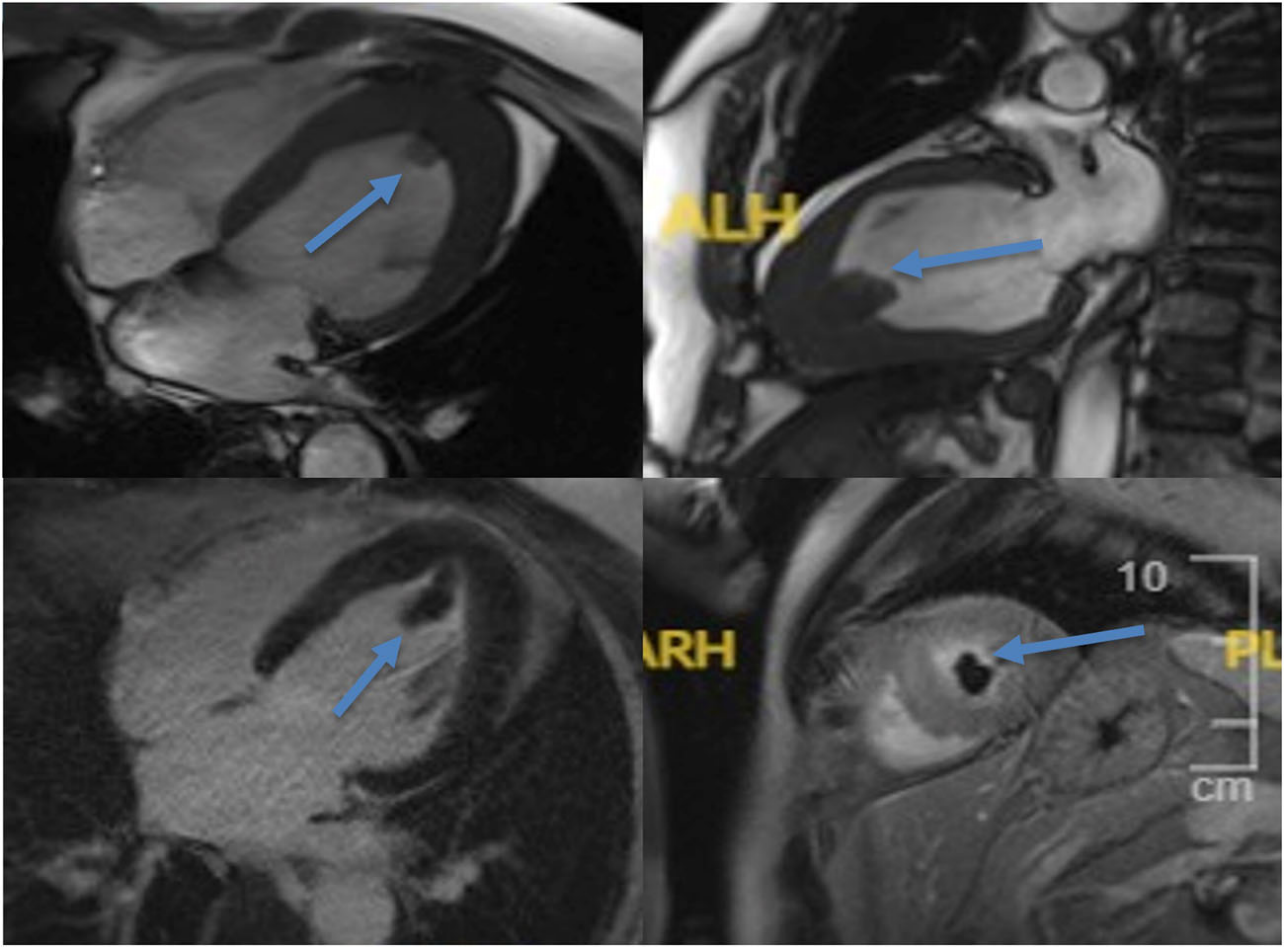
Cardiac magnetic resonance imaging demonstrating a large left ventricular (LV) apical thrombus in a patient with apical hypertrophic cardiomyopathy (ApHCM). (Top left and top right): Steady‐state free precession (SSFP) cine images in long‐axis views show a well‐demarcated apical mass (arrows) within a hypertrophied LV apex. (Bottom left): Early post‐contrast inversion recovery image demonstrates lack of enhancement of the apical mass, consistent with thrombus (arrow). (Bottom right): Late gadolinium enhancement (LGE) image further confirms the non‐enhancing nature of the apical thrombus (arrow), with surrounding myocardial enhancement indicating apical fibrosis. No apical aneurysm is evident. [Color figure can be viewed at wileyonlinelibrary.com]
